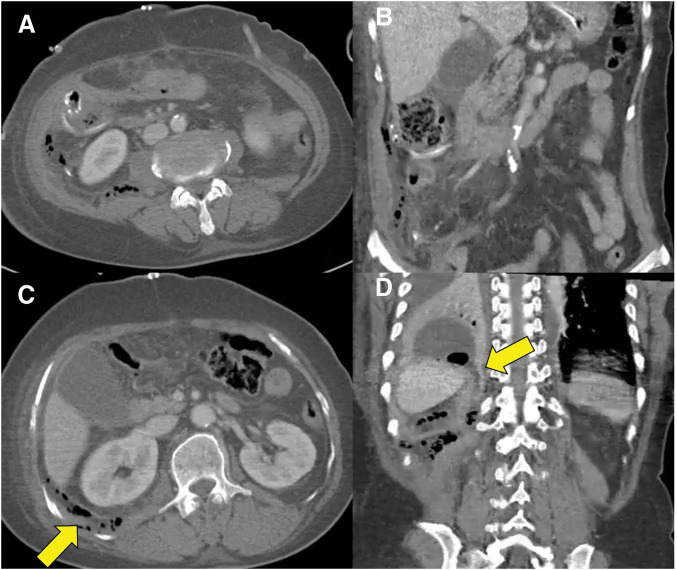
Postoperative CT shows small air-containing fluid near the hemicolectomy site (A and B). Empyema with suspected pleuro-retroperitoneal fistula is seen (yellow arrows) (C and D).
